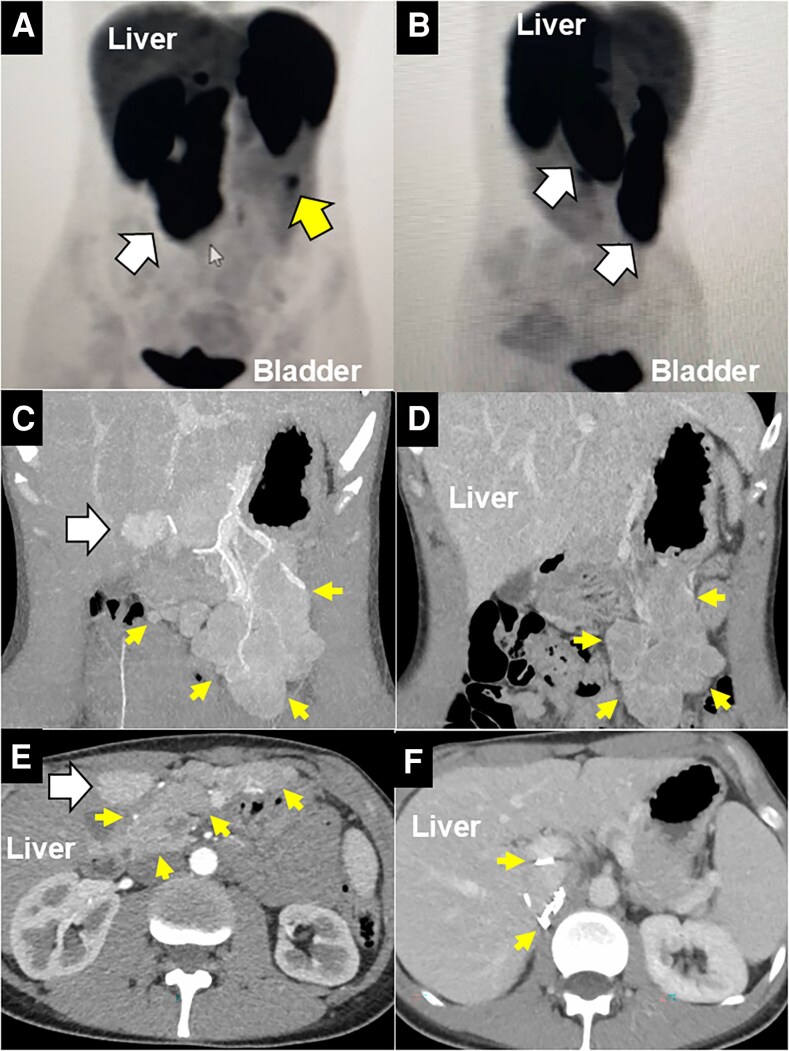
Preoperative imaging demonstrating massive pheochromocytomatosis. A-B, ^68^Ga-DOTATATE PET scan performed at the patient's home institution, displaying large areas of intra-abdominal uptake (white arrow, A coronal, B sagittal views) in the right upper quadrant, as well as smaller areas on the left side (yellow arrow). Physiological uptake is noted in the liver and urinary bladder. C-D, Coronal CT images of arterial (C) and venous phase (D) demonstrating numerous multi-loculated, highly vascular intra-abdominal lesions (mainly in the omentum and in the right adrenalectomy bed; yellow arrows), and one solitary liver lesion in segment IV (white arrow). E-F, Axial CT images of arterial (E) and venous phase (F) demonstrating numerous multi-loculated, highly vascular intra-abdominal lesions (yellow arrows), and one solitary liver lesion in segment IV (E, white arrow), and, more cephalad, evidence of numerous clips (F) and tumor deposits in the right adrenalectomy bed (yellow arrows). All CT scan images depicted are according to a modified adrenal protocol of the Carling Adrenal Center and Hospital for Endocrine Surgery.
